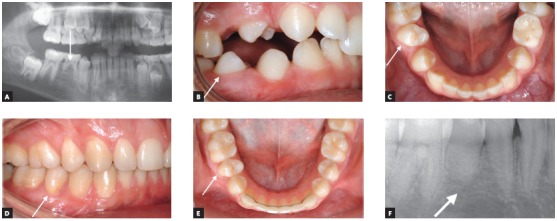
Congenitally missing lower second premolar on the right side was seen during radiographic examination of an 11-year-old boy with Class II division 2 malocclusion and maxillary crowding (A). The roots of the primary second molar at the site of the missing premolar were short. The treatment plan included transplantation of the developing upper right second premolar (arrow) to the position of the congenitally missing mandibular premolar, followed by the fixed appliance treatment to close the extraction space and establish normal overjet and overbite. The transplanted premolar (arrow) erupted spontaneously in the oral cavity six months after transplantation from the subgingival position after surgery (B, C). The fixed appliances were bonded two years after transplantation and eruption of all permanent teeth. Normal tooth contacts were established after orthodontic treatment and overjet and overbite were corrected (D, E). Pulp obliteration and no hard tissue pathology were present at the transplanted premolar (F).
